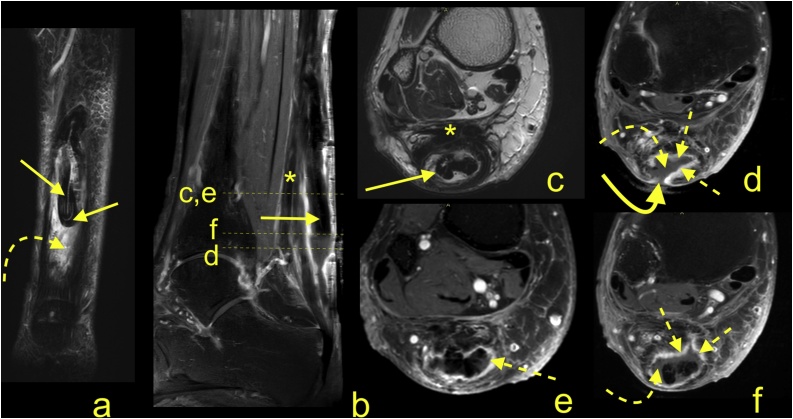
Rupture and infection of the Achilles tendon graft. A 76-year-old patient received a tendon graft because of a chronic Achilles tendon rupture 6 months ago. She had a wound that would not heal, despite treatment with negative pressure wound therapy. Fever and malaise in the past few days and worse function of the reconstructed tendon. MRI (a-f) with contrast was performed. Rupture of the graft with retraction was revealed (straight arrow). Fluid collection with enhanced walls (dashed arrows) around the graft and fistula (curved arrow) is visible. Extensive thickening of the paratenon (*) and adhesions in Kager's fat pad are present. A- T2- weighted with fat suppression, b- T1- weighted with fat suppression and contrast, c- T1- weighted, d, e and f - T1- weighted with fat suppression and contrast.
